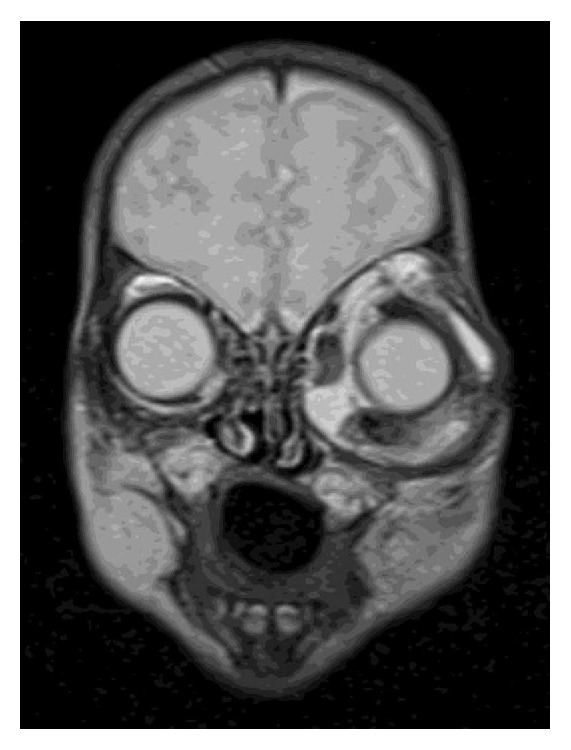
CT scan of initial presentation shows the large cystic mass was surrounding the globe and stretching the extraocular muscles in the left orbit. The right eye appears normal.
